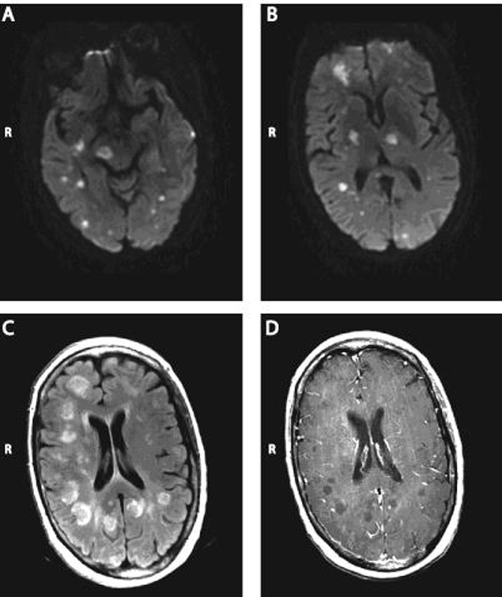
Axial MR images demonstrating diffusion restriction (A & B), hyperintensities on FLAIR images (C), and hypointensities on T1 images (D).
